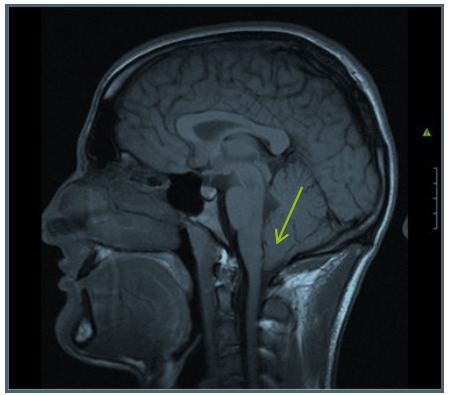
MRI of the brain (Day 17)
